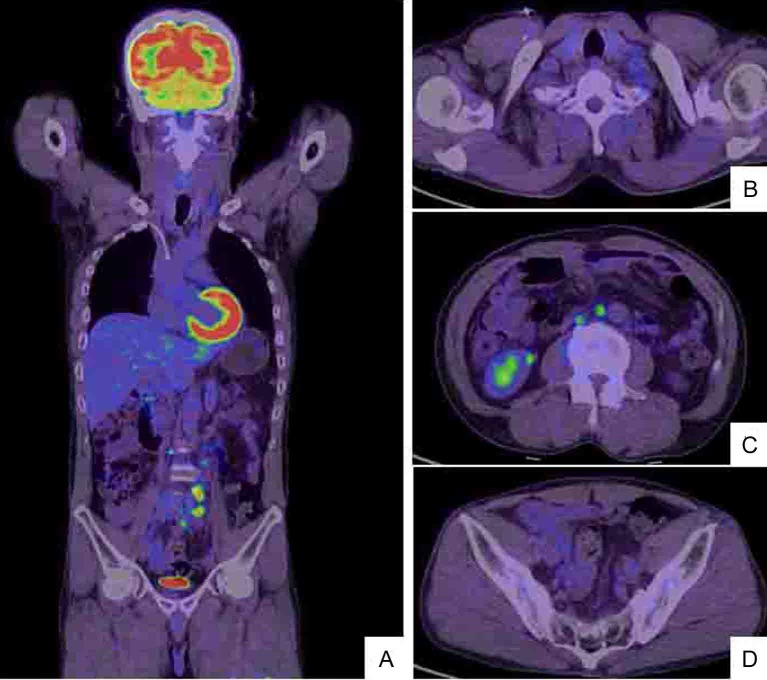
After 6 courses of the chemotherapy (the combination of mFOLFOX6 and bevacizumab), whole body PET/CT scan (A) demonstrates that the left cervical metastatic LNs (B) and the abdominal periaortic LNs are reduced in size and the intensity of hot lesions is obviously decreased (C). The regional colic LNs (D) are not recognized as hot lesions.
